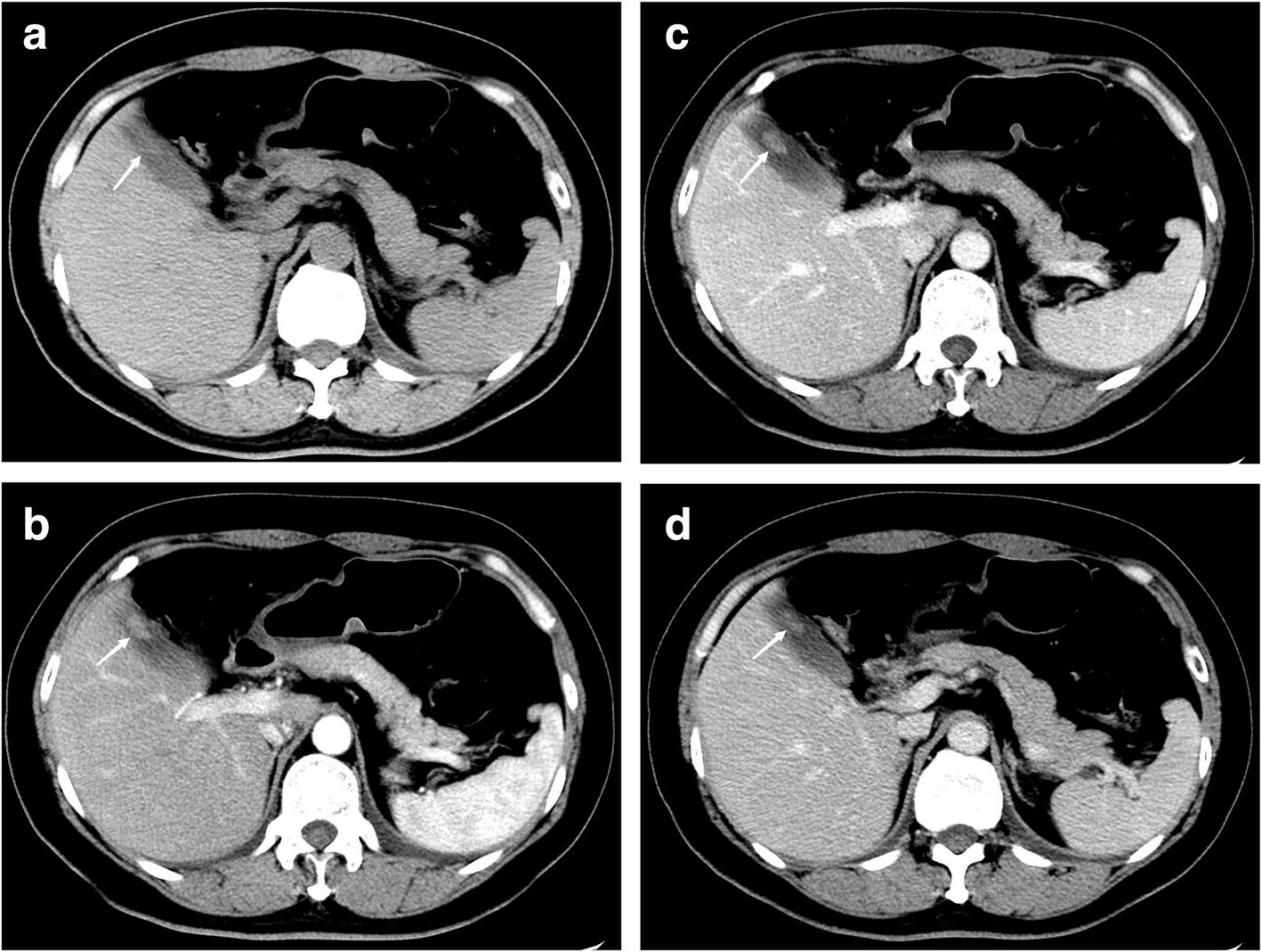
CT images of a 50-year-old woman with benign gallbladder polypoid lesion (gallbladder adenoma), which was narrow base. a Plain phase CT values was 36 HU, b artery phase CT values was 75 HU, c portal venous phase CT value was 74 HU, and d delayed phase CT value was 60 HU
